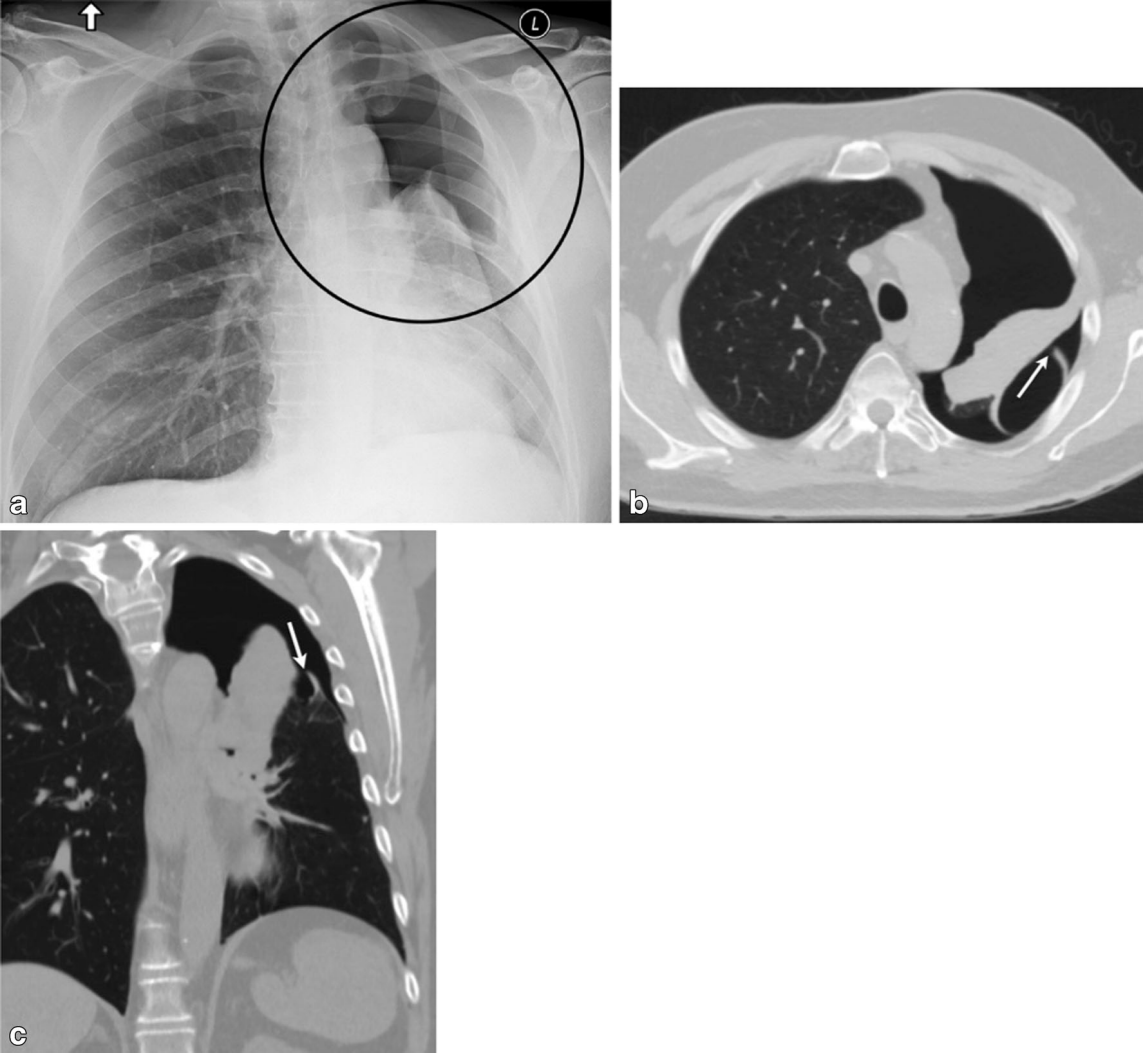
A 60-year-old male with left upper lung carcinoma; post radiation therapy. Frontal chest radiograph (a) demonstrates a left apical pneumothorax (*circle*). Axial (b) and coronal (c) CT images demonstrate discontinuity of visceral pleura (*arrow*) with associated alveolopleural fistula
